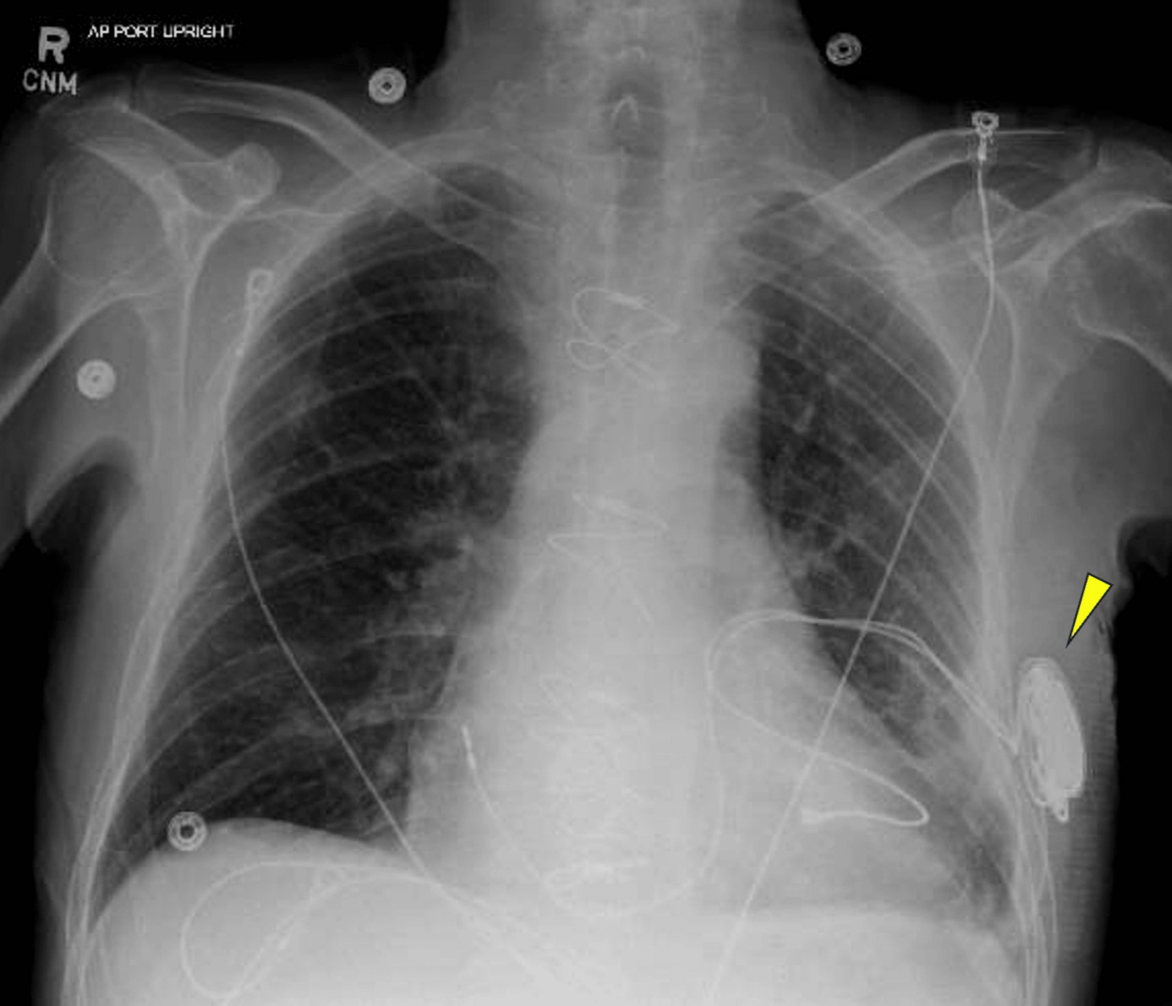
X-ray of the newly placed leads on the epicardium to a new pacemaker beneath the left axilla, away from the original pocket.
